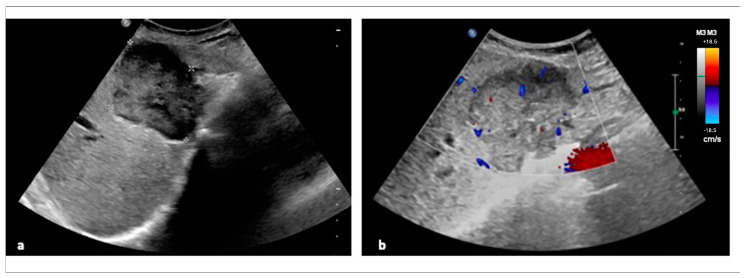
Ultrasound features of iCCA. Ultrasound features of iCCA in a 79-year-old female: (a) a hypoechoic mass with polylobed margins occupying the IV hepatic segment. (b) Signs of perilobular and intranodular vascularization on Color Doppler examination. Abbreviation: iCCA, intrahepatic cholangiocarcinoma.
